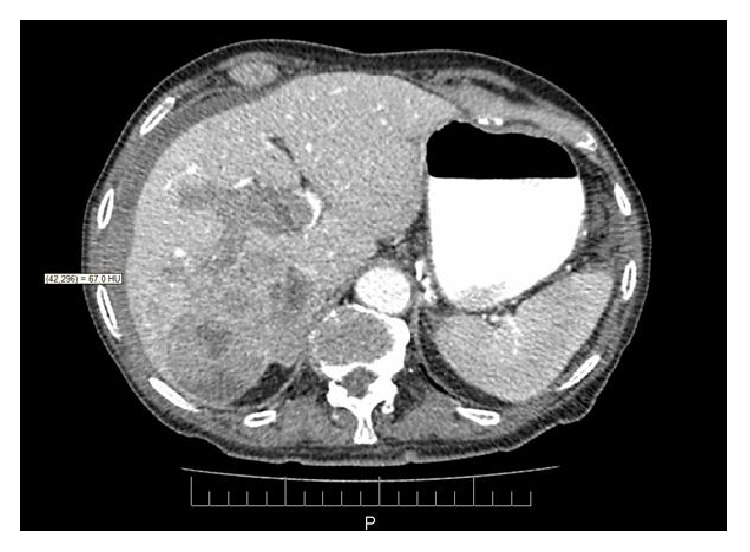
Abdomen CT showing a 7.5 cm mass occupying the right lobe of the liver, thrombosis of the right portal vein, and hemoperitoneum (HU = 67).
